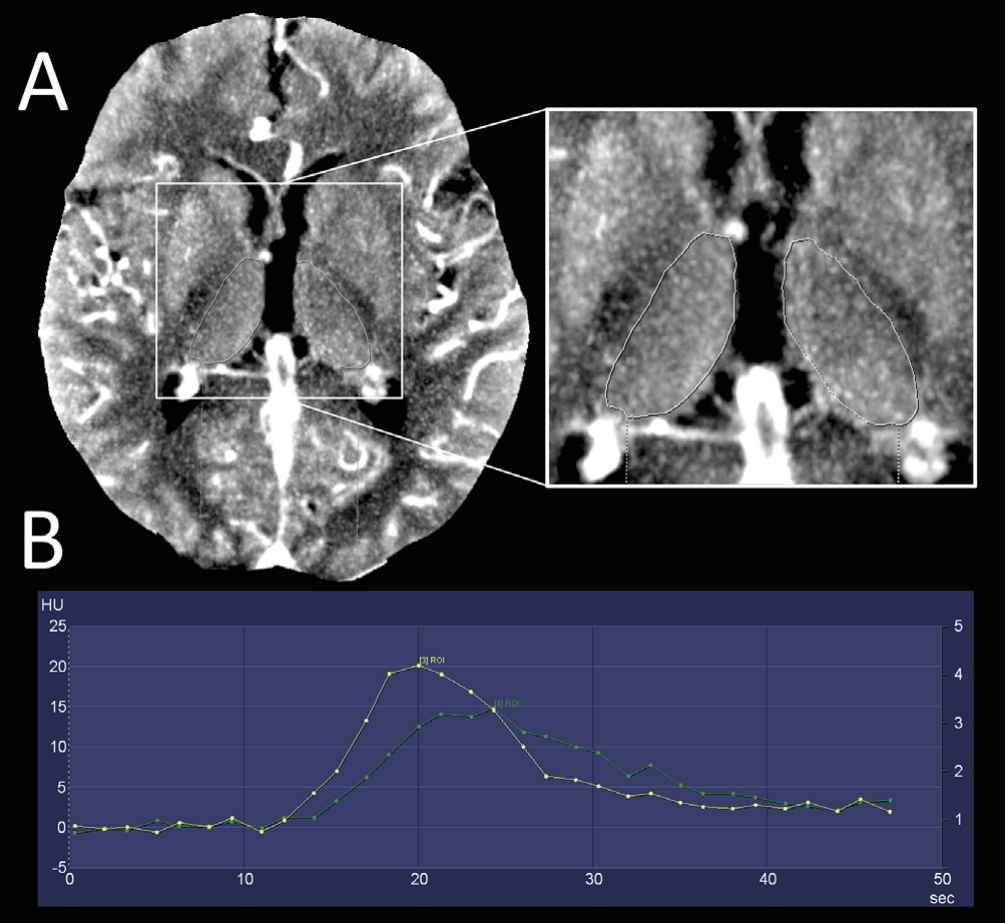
Measurements of thalamic perfusion parameters on temporal MIP CTP images. A) Example of manual segmentation of both thalami in a patient with left M1 occlusion. Right: Enlarged section with segmented thalamic outline (white) on both sides. B) Derived tissue attenuation curves of ipsilesional ROI (green) and contralesional ROI (yellow). Abbreviations: MIP, maximum intensity projection; CTP, CT perfusion; ROI, region of interest; HU, Hounsfield units. (For interpretation of the references to colour in this figure legend, the reader is referred to the web version of this article.)
